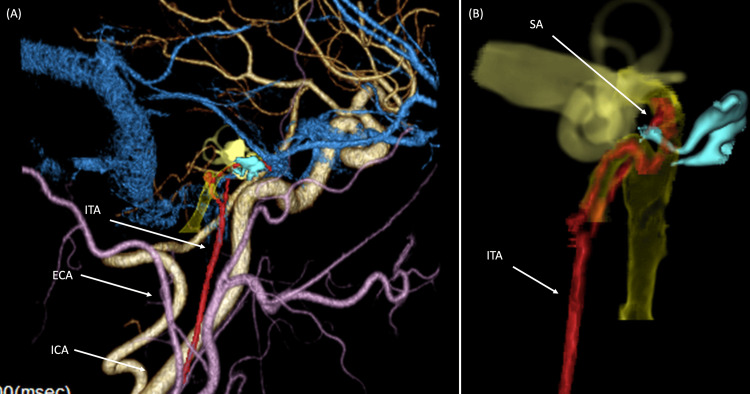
CTA images. (A) This image shows the location of the ear ossicles and surrounding vascular structures. The inferior tympanic artery doesn't divide from the internal carotid artery but the external carotid artery. (B) The stapedial artery running between the anterior and posterior limbs of the stapes. ITA: Inferior Tympanic Artery; ICA: Internal Carotid Artery; ECA: External Carotid Artery; SA: Stapedial Artery Image credits: Yoshiyuki Sasano
